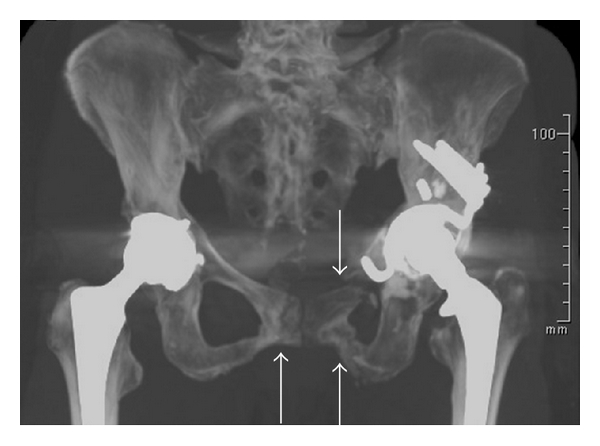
CT image showing pelvis. Arrows indicate fracture site of pubic rami.
